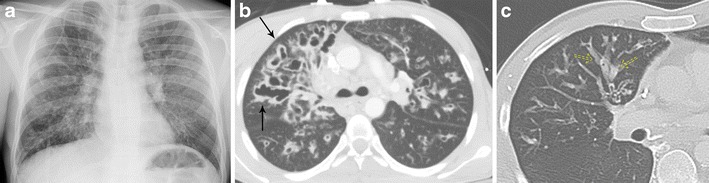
Bronchiectasis due to cystic fibrosis. a Frontal chest x-ray demonstrating upper lobe prominent course interstitial markings, patchy areas of increased airspace opacity, and bronchiectasis involving the lungs bilaterally. b Axial CT imaging demonstrating upper lobe-predominant cystic and varicoid bronchiectasis and bronchial wall thickening (*solid arrow*). c Axial CT imaging in another patient demonstrates bronchiectasis and mucoid impaction (*dashed arrow*) with “finger-in-glove” sign
